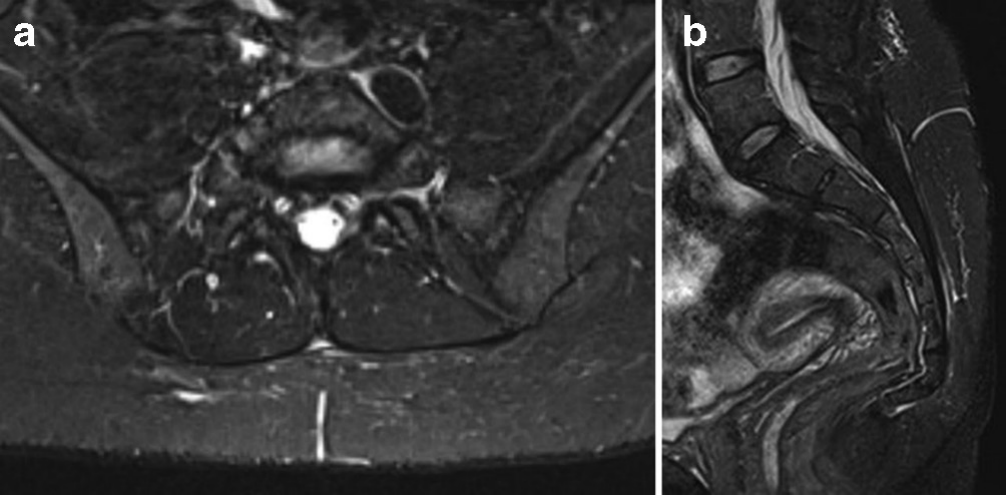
(a) Axial and (b) sagittal short tau inversion recovery (MRI) of sacrum post-sclerotherapy showing no recurrent fluid collection. Trace increased signal at site of previous lesion is likely post-procedural in nature. Sagittal sequence confirms resolution of distal extension of lesion also.
